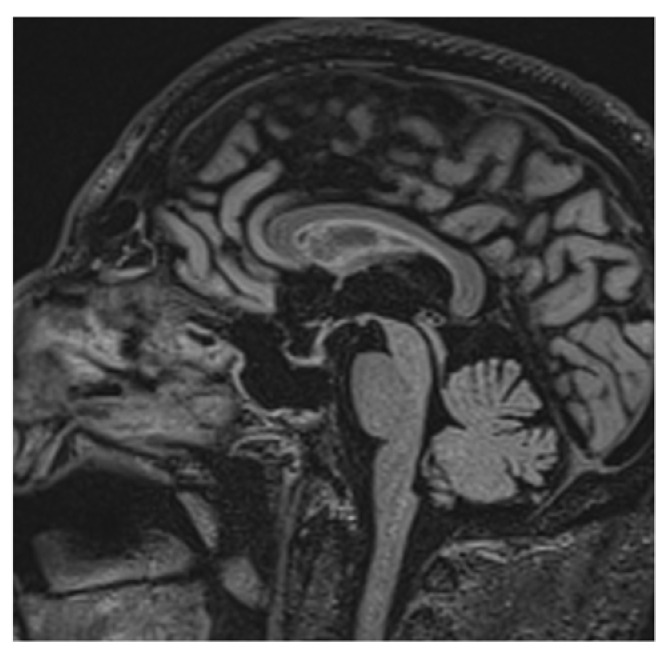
Partial “empty sellae syndrome” on brain MRI coronal section.
